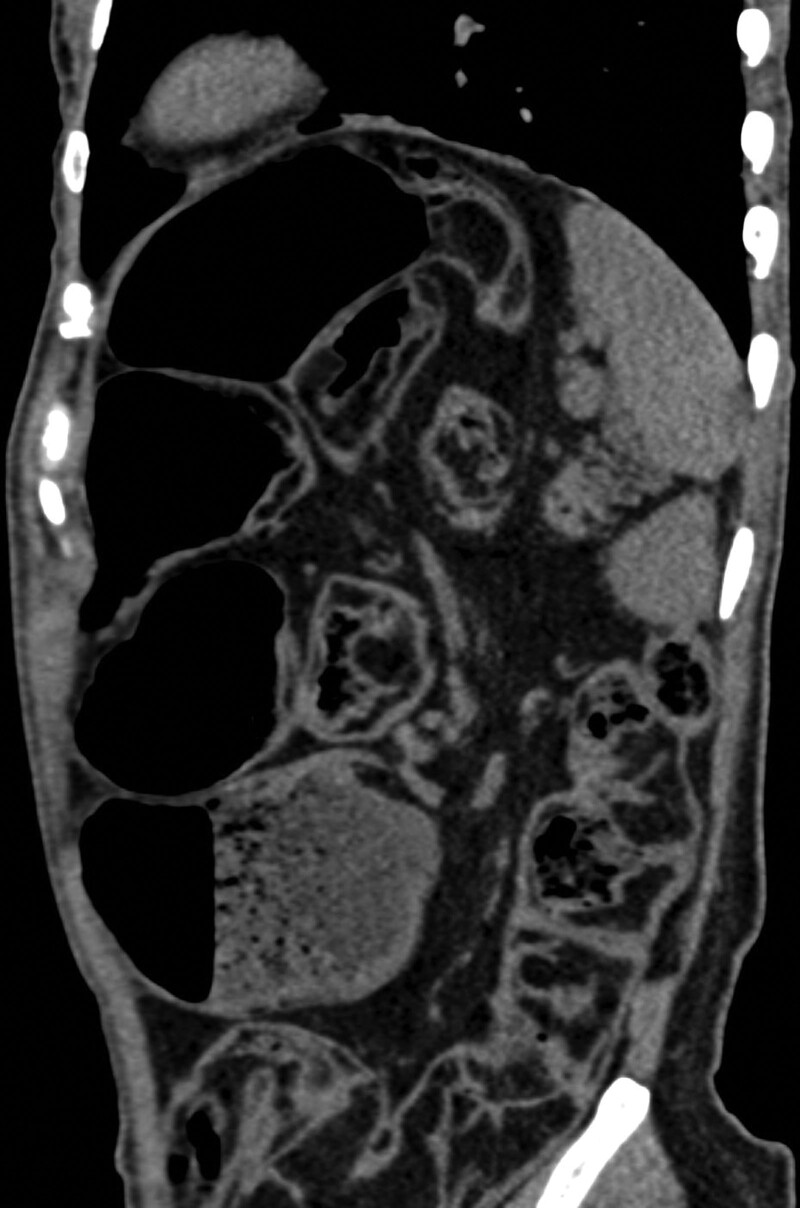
Coronary CT showed diffuse nodular submucosal lipomatous changes and significant intestinal dilatation.
